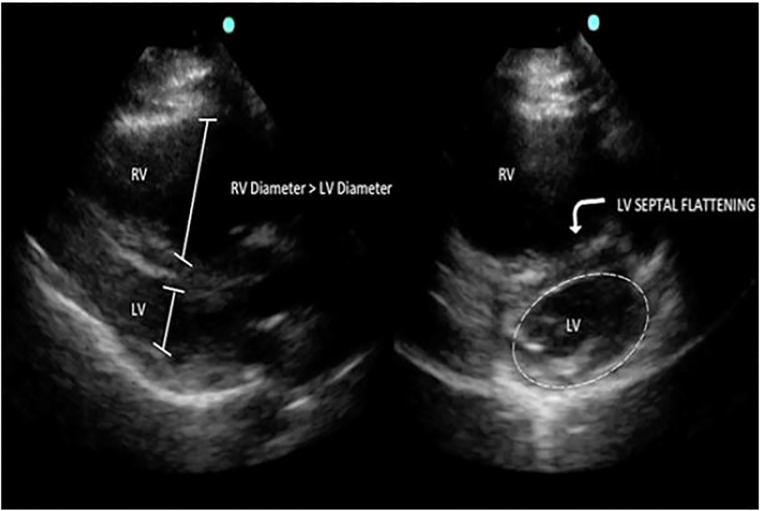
Point-of-care cardiac ultrasound performed pre-thrombolysis. Parasternal long axis view (left) and parasternal short axis view (right) demonstrating right heart strain. *LV*, left ventricle; *RV*, right ventricle.
